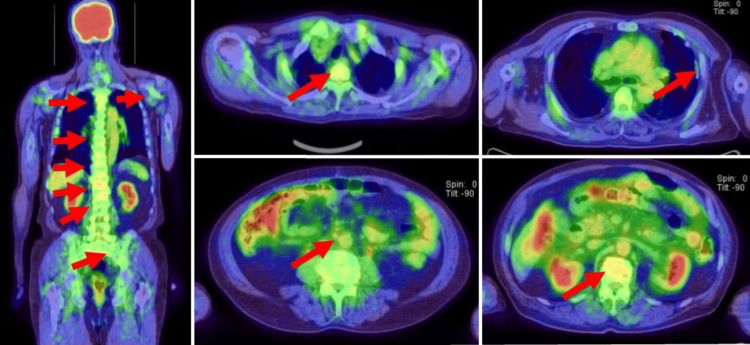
The status of multiple metastases after the administration of pembrolizumab. After two courses of pembrolizumab, the patient's symptoms remarkably improved and the abnormal systemic accumulation on ^18^F-fluorodeoxyglucose positron emission tomography-computed tomography almost disappeared (red arrowheads).
